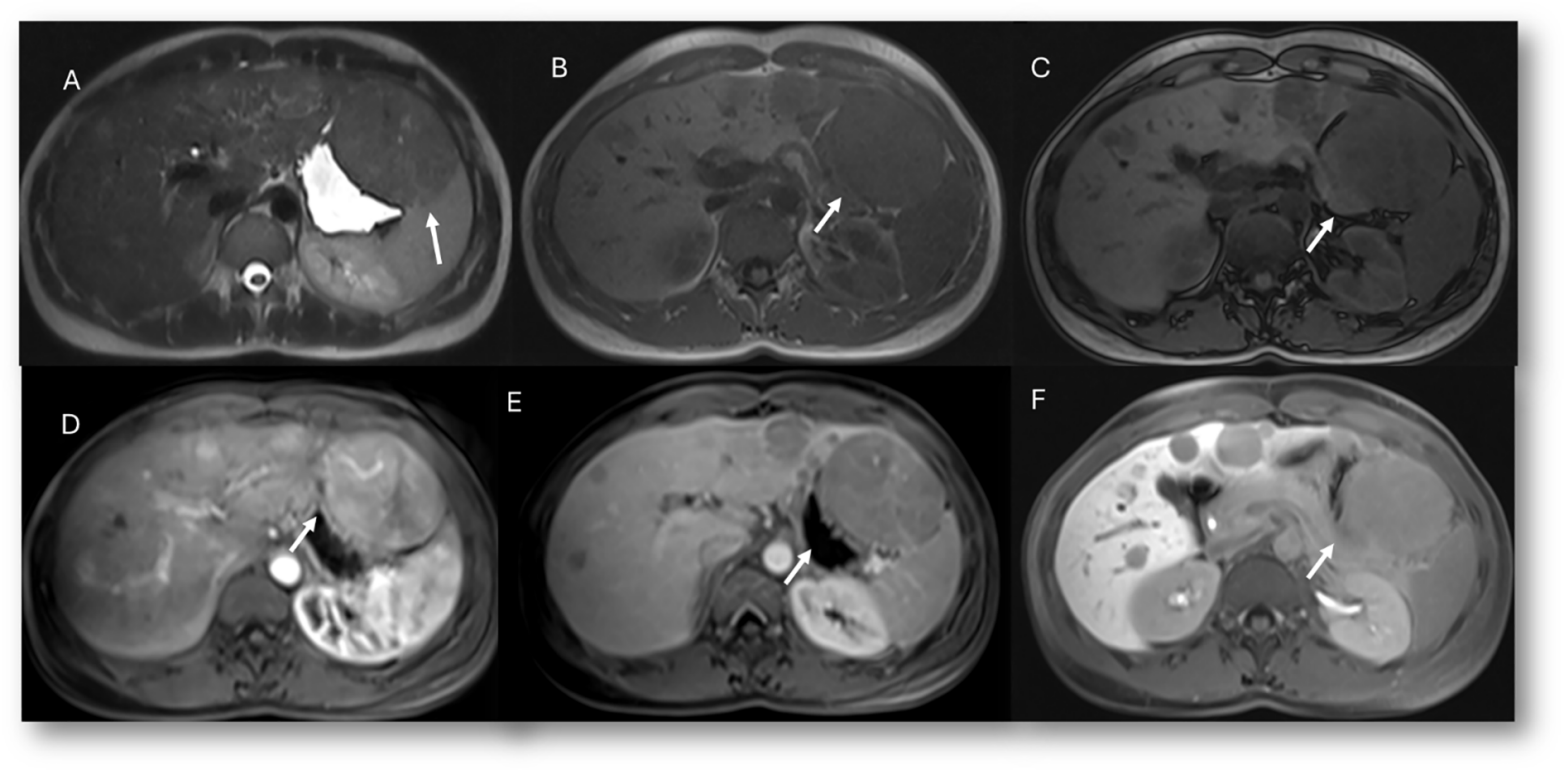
MRI assessment of multiple adenoma lesions (arrows) with Gd-EOB-DTPA contrast. In (A) (T2-W sequence), lesions are hyperintense; in (B) (T1-W in-phase) and (C) (T1-W out-of-phase), lesions are hypointense. During contrast study, lesions show non-rim hyperenhancement during arterial phase (D), washout during portal phase (E), and hypointense signal on hepatospecific phase (F).
